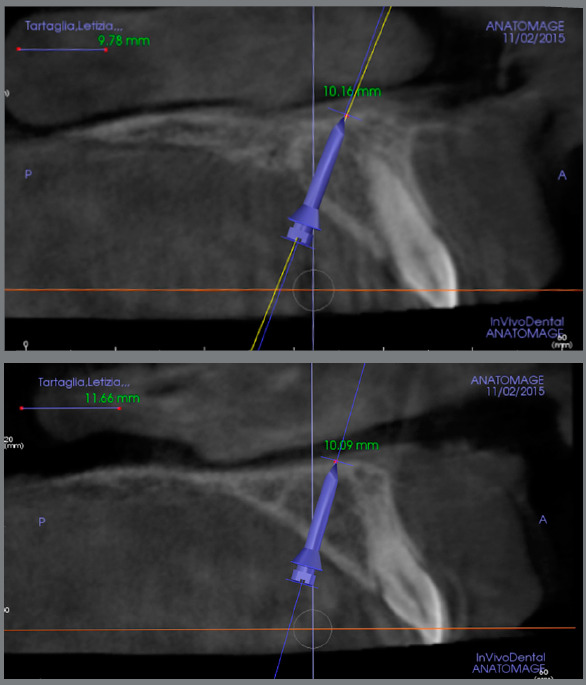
Digital planning of the mini-implants insertion by matching the DICOM files of the CBCT and the STL files of the digital models, according to the MAPA protocol.
